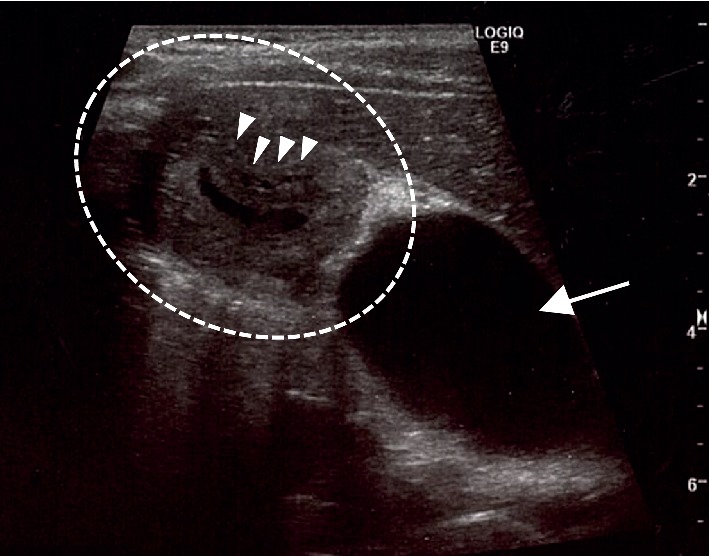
Repeated ultrasonography the next day of admission revealed that the proximal dilated gallbladder (arrow) was continuous with the mass (circle), the wall of which contained several small cysts (arrowheads).
